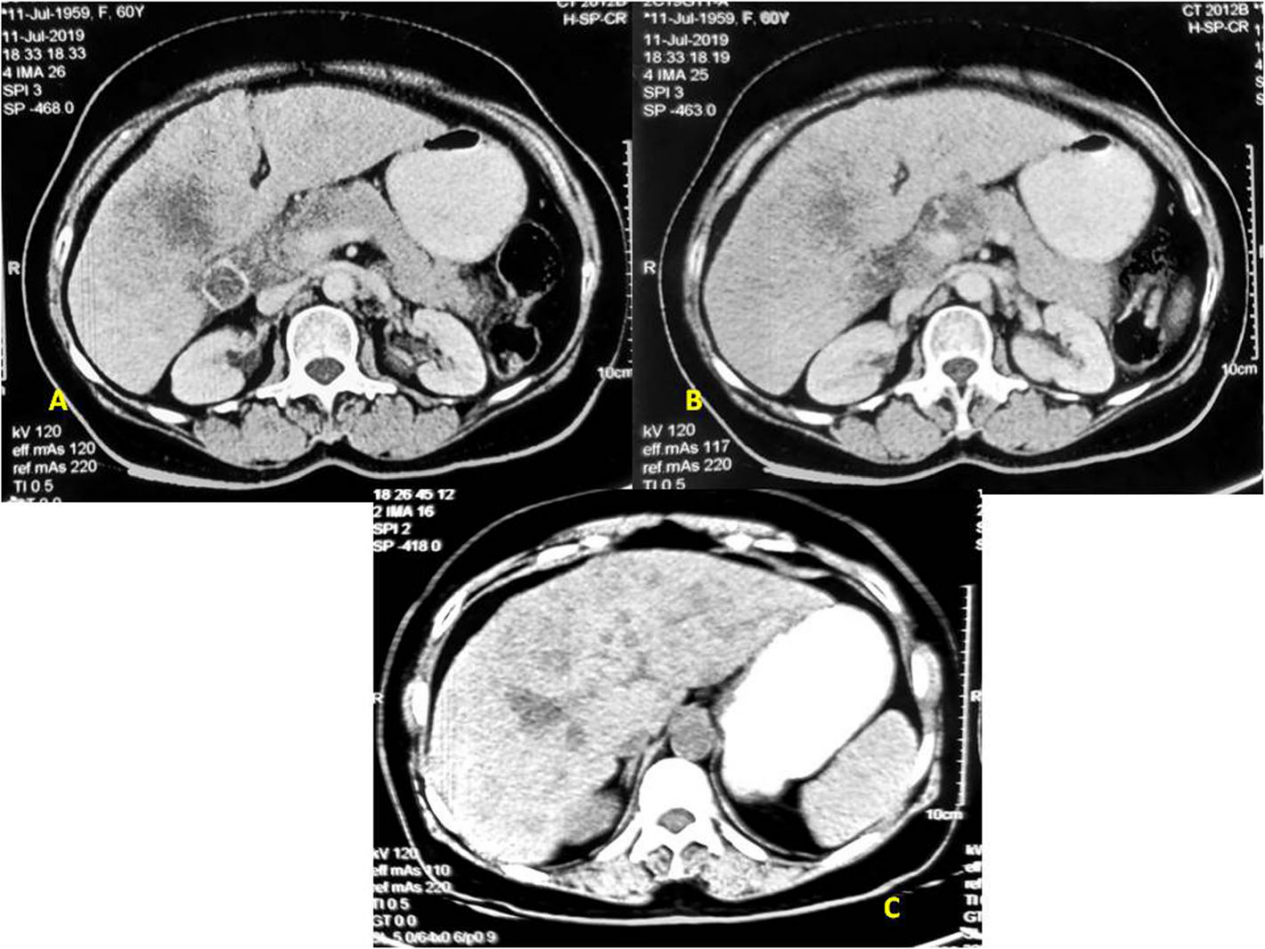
Pretreatment CT scan showing a mass in the fundus of gallbladder infiltrating IVB, V segment of liver with gallstones. b Liver metastasis with periportal and precaval lymphadenopathy. c Extensive liver metastasis
